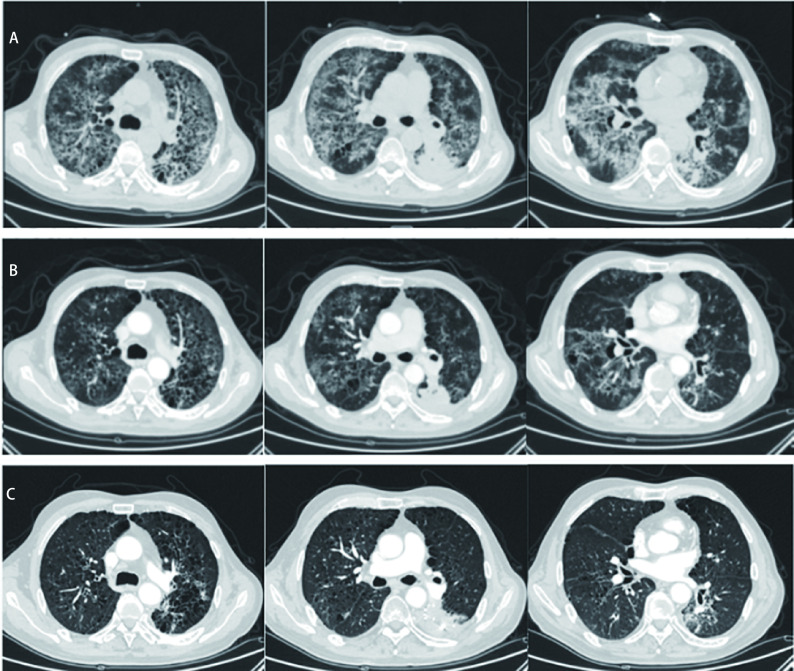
CIP治疗前后胸部CT。A：治疗前胸部CT（2019年10月28日）；B：糖皮质激素大剂量冲击治疗后胸部CT（2019年11月18日）；C：吡非尼酮治疗8月余后胸部CT（2020年7月14日）； Chest CT before and after treatment of CIP. A: Chest CT before treatment (October 28, 2019); B: Chest CT after high-dose glucocorticoid pulse therapy (November 18, 2019); C: Chest CT after pirfenidone treatment for more than 8 months (July 14, 2020).
